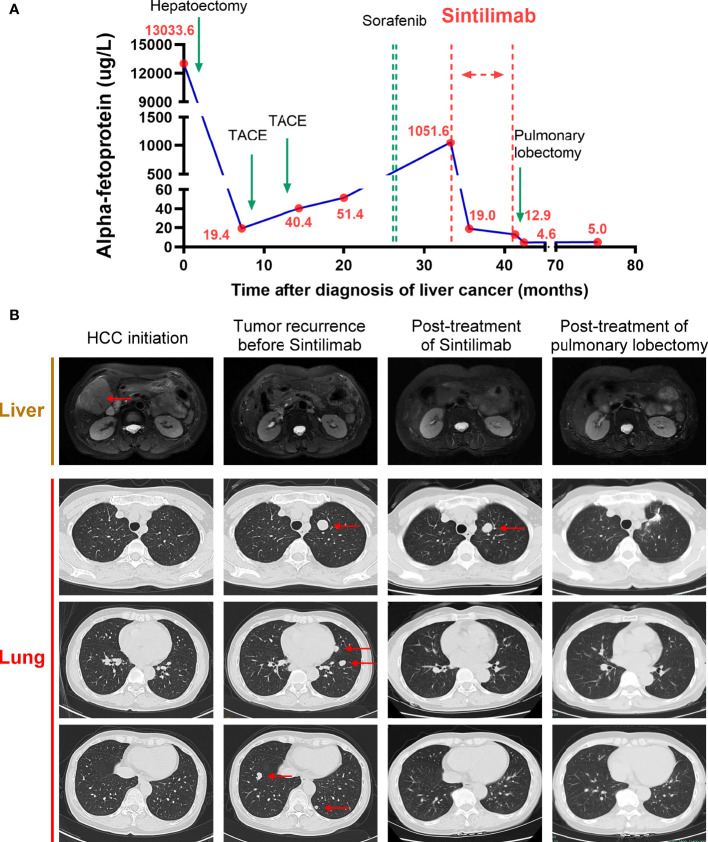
Course of the patient’s treatments. (A) Timelines of main treatments and changes in α-fetoprotein (AFP) levels during each period.(B) Representative imaging assessments during each period, including hepatic magnetic resonance (T2-weighted) and pulmonary computerized tomography (CT) in three different sections. Red arrows indicate the tumors.
